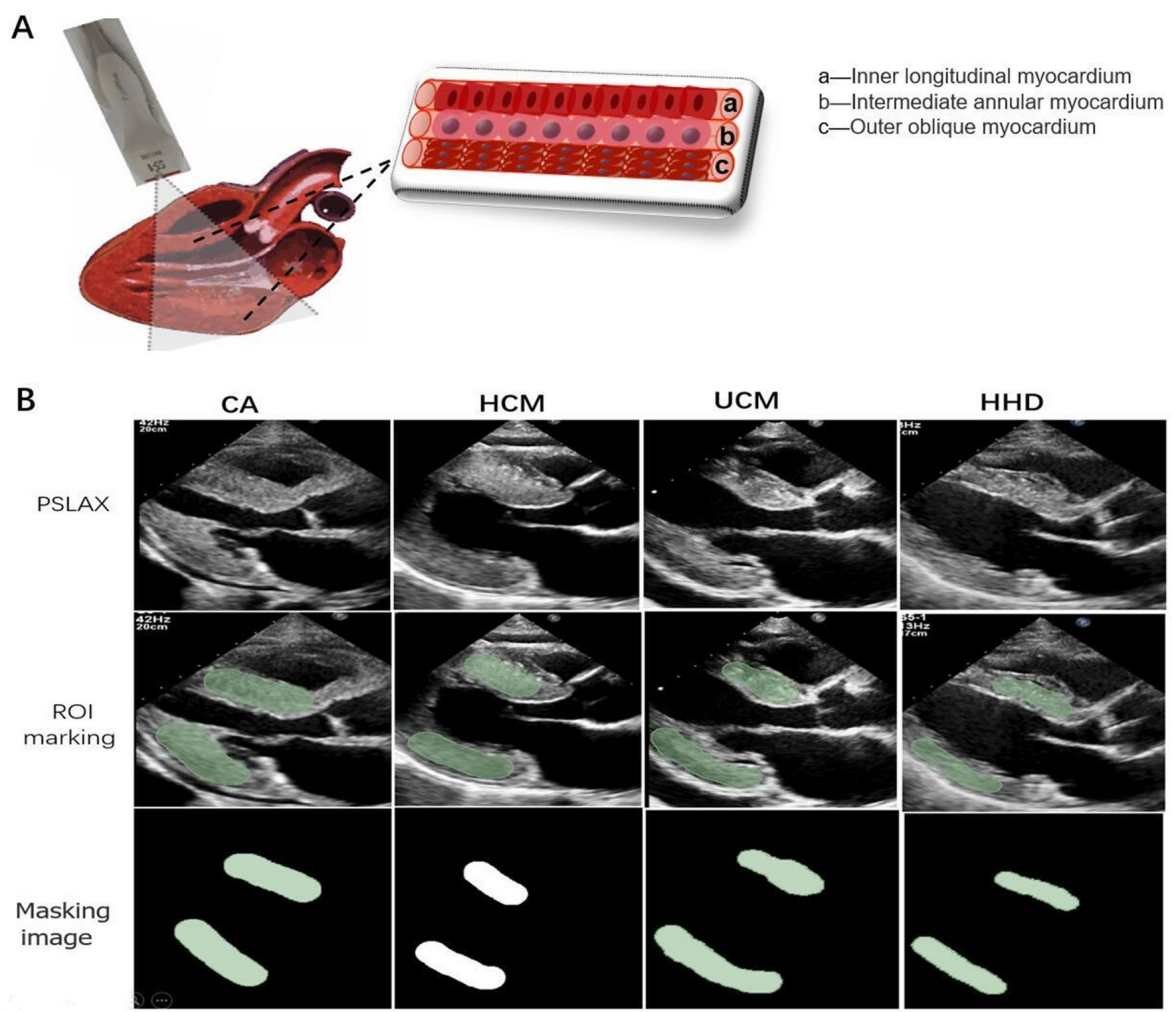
Myocardial texture feature extraction based on two-dimensional echocardiography. A Sketch map of PLAX section of left ventricle; B The region of interest (ROI) segmentation of the cardiac ultrasound image. PSLAX, parasternal long axial section of the left ventricle. ROI marking, marking of interventricular septum and posterior wall of left ventricle as region of interest. Masking image, Image binarization is performed on the extracted mask
